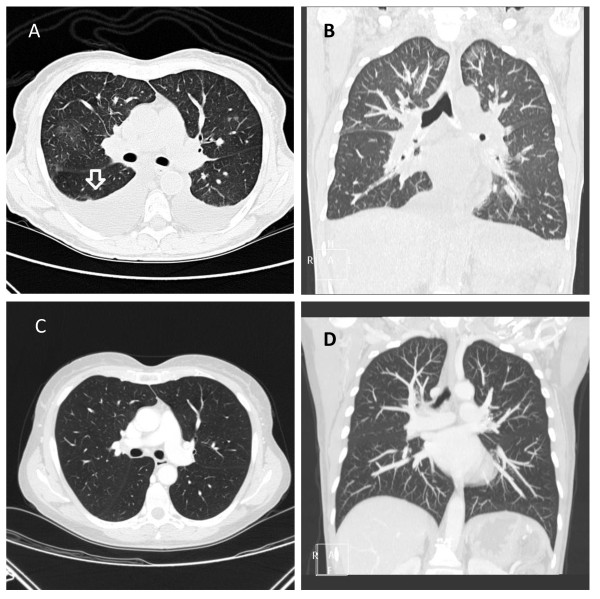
Chest CT scan showing evidence of pulmonary edema (A, axial plane – B, coronal plane) and disappearance of pleural effusion and pulmonary edema (C-D).
